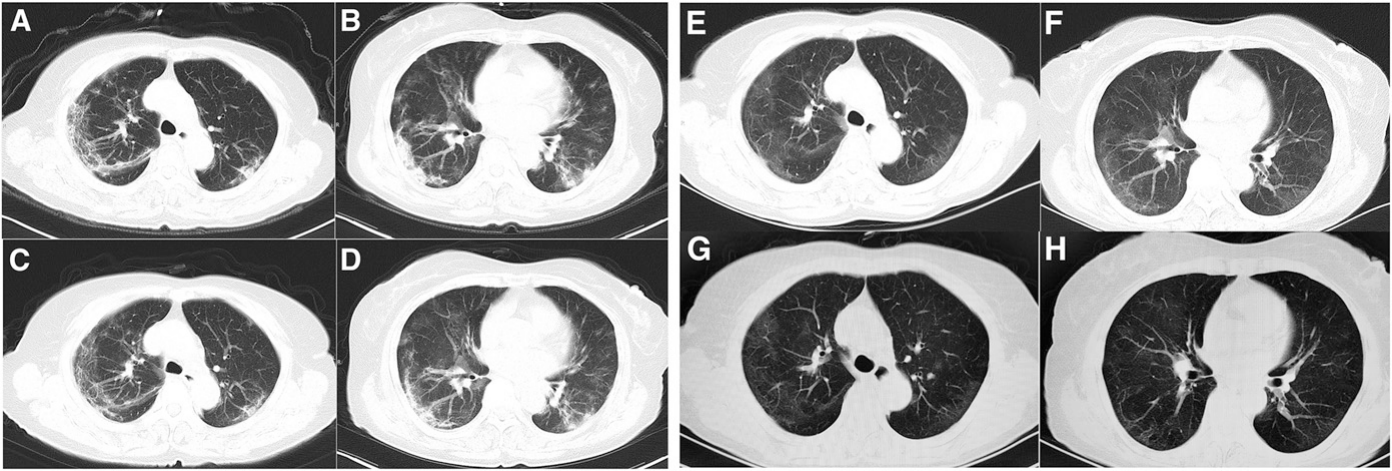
Chest computed tomography images. The images show multiple patchy shadows and bilateral subpleural ground-glass opacities and infiltrates, with improvement over time. (A and B): February 11; (C and D): February 17; (E and F): March 6; (G and H): March 21 (illness day 15, 21, 39, and 54, respectively).
